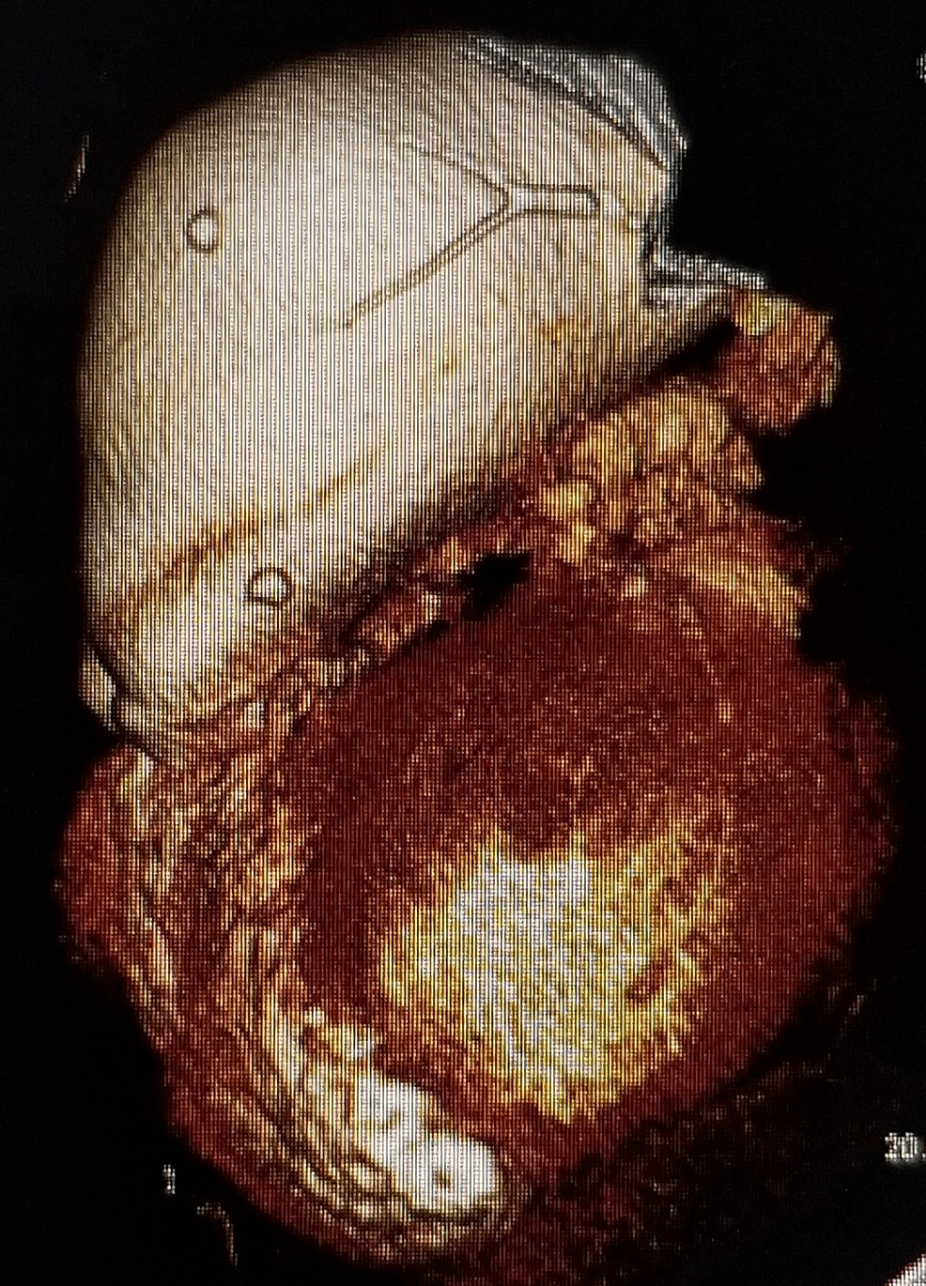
Severe PAA as visualized on 3D CTA
